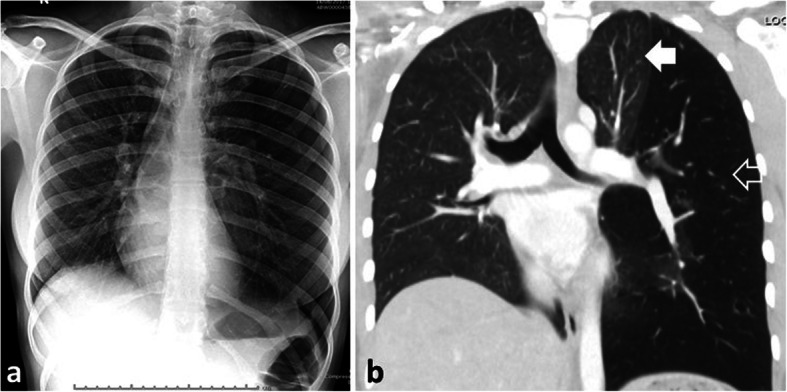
a Chest radiograph showing hyper-inflation of the left lung with shift of the mediastinum to the right and downward displacement of the left hemi-diaphragm. b Chest CT scan, coronal view, showing hyper-inflation of the left lower lobe (outlined arrow) with compression of the upper lobe (white arrow)
